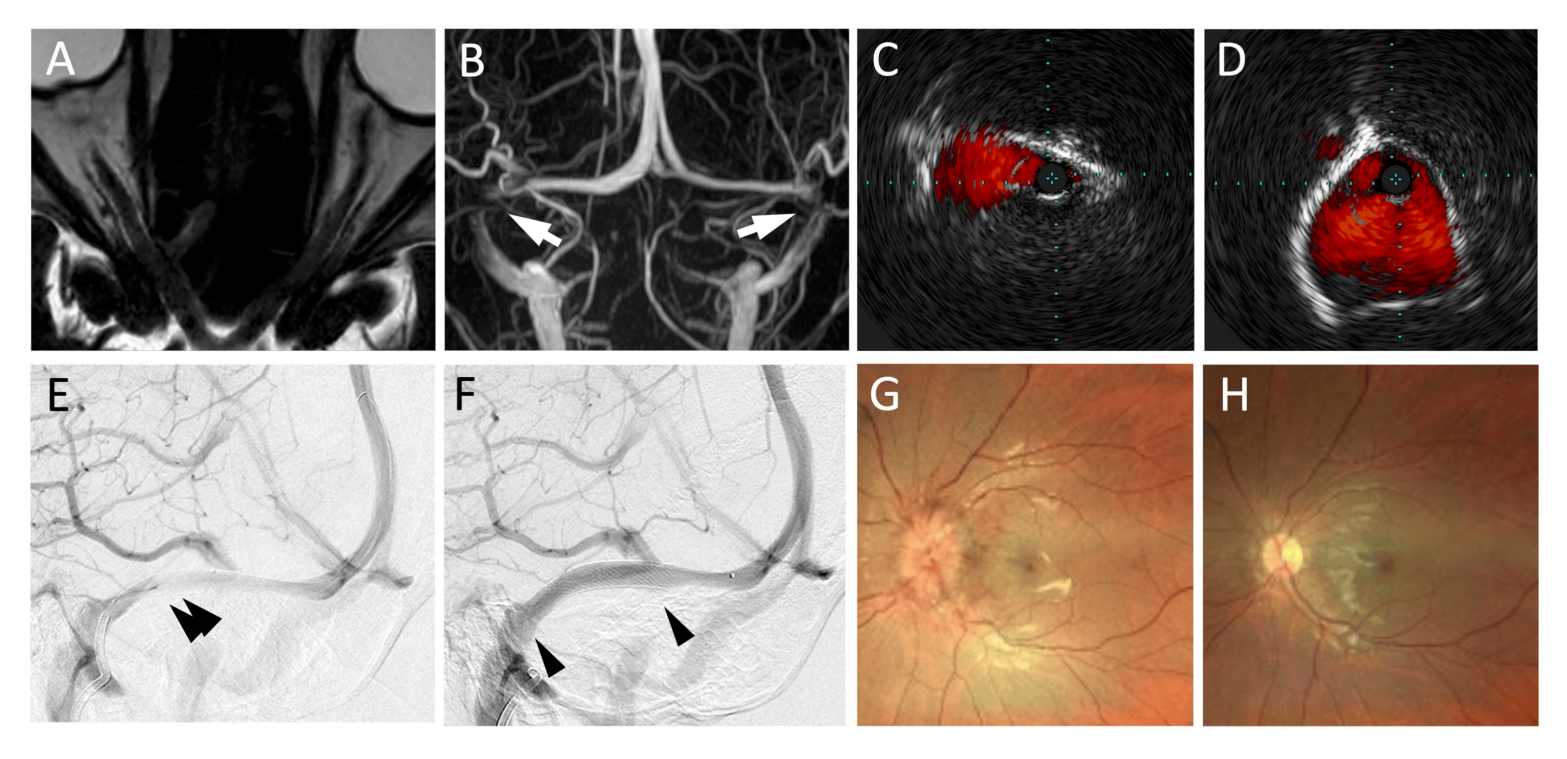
Images from Case 1 (A) MRI on admission revealed optic nerve tortuosity, enlargement of the peripapillary subarachnoid space, and flattening of the dorsal sclera of the eyes. (B) MRI venography showing bilateral stenosis of the transverse sinuses (arrow). (C) IVUS image shows the triangular extrinsic stenosis at the transverse-sigmoid sinus junction. (D) IVUS image provides precise measurements of the medial part of the transverse sinus, helping determine the stent size. (The distance between blue dots represents 1 mm.) (E) Right internal cerebral angiogram demonstrated transverse sinus stenosis (double arrow). (F) Angiogram after stenting exhibited the dilatation of the transverse sinus. The stent (between the two arrowheads) did not disturb the flow in the vein of Labbé, although it covered the vein’s orifice. (G)-(H) Ophthalmoscopic examination revealed severe papilledema on admission (G) and its recovery on postoperative day 7 (H). MRI: magnetic resonance imaging, IVUS: intravascular ultrasonography.
